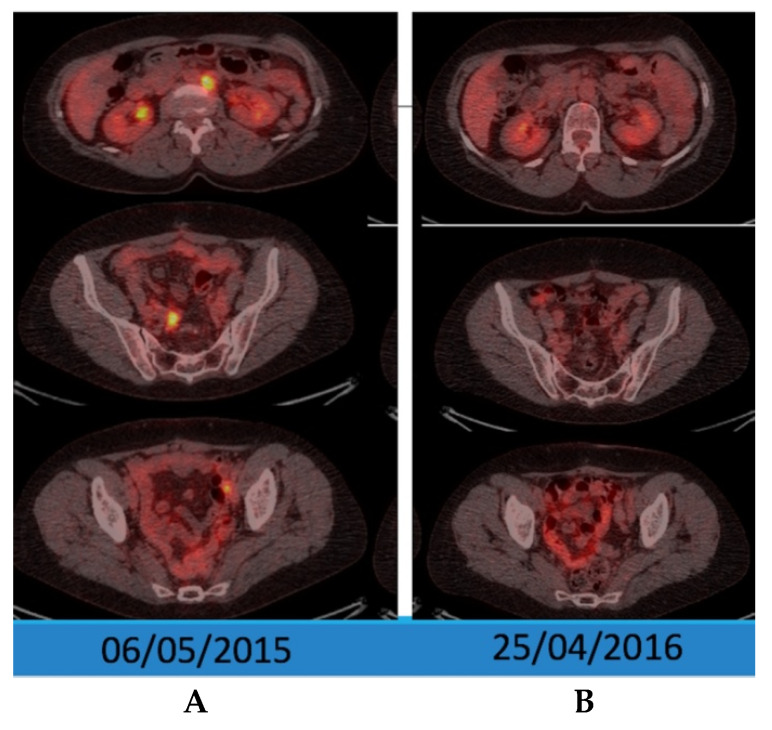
F18-FDG PET/CT scans—2015, before chemotherapy and 2016, after treatment, evaluating the therapy response: axial section abdominal level (upper images), pelvic level with right lymph node (middle image), and pelvic level with left lymph node (lower image), showing tracer high uptake in left abdominal latero-aortic lymph node (SUVlbmMax—5.1), and in bilateral pelvic lymph nodes ((B) SUVlbmMax—4.8 and (A) SUVlbmMax—3.9), with complete remission in 2016 on all sites.
